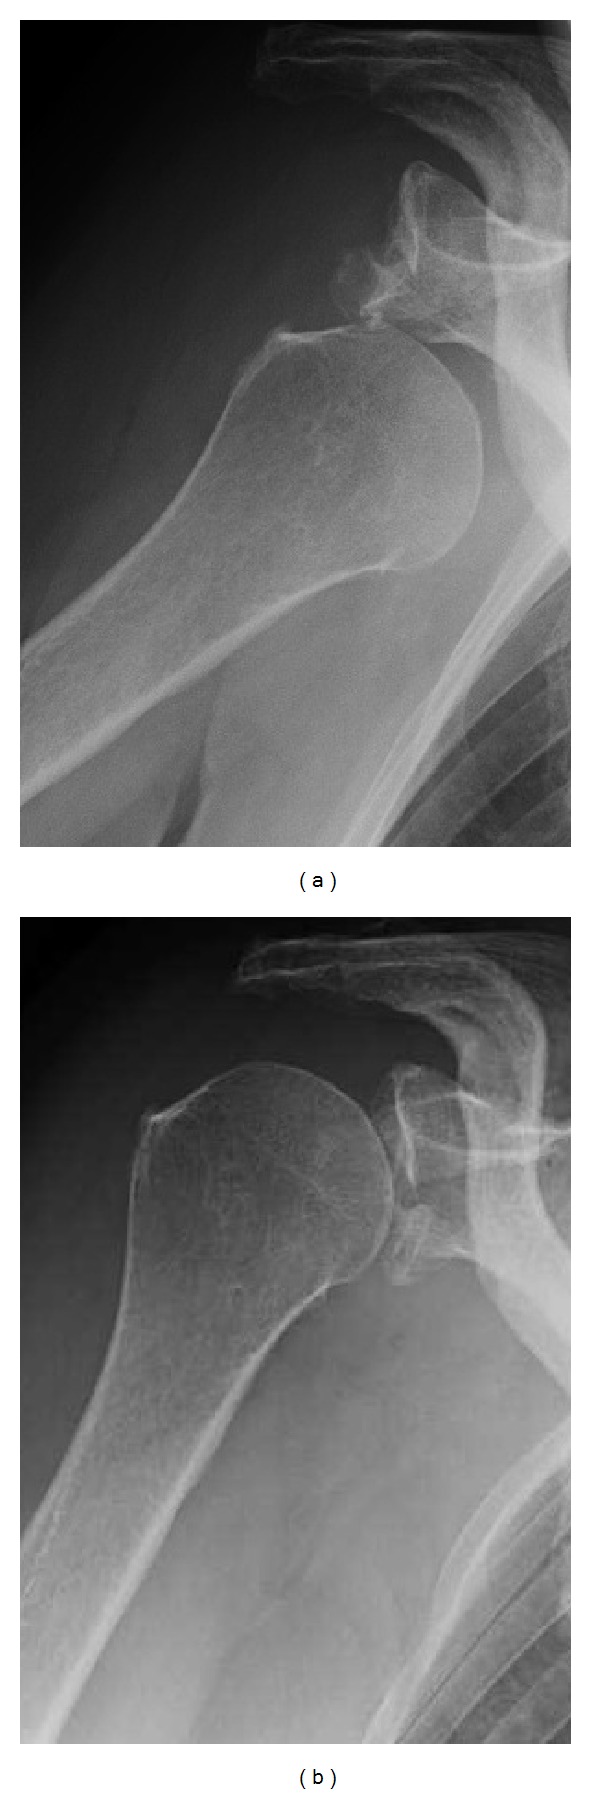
(a) X-ray imaging at the time of injury. (b) X-ray imaging after reduction of anterior shoulder dislocation.
